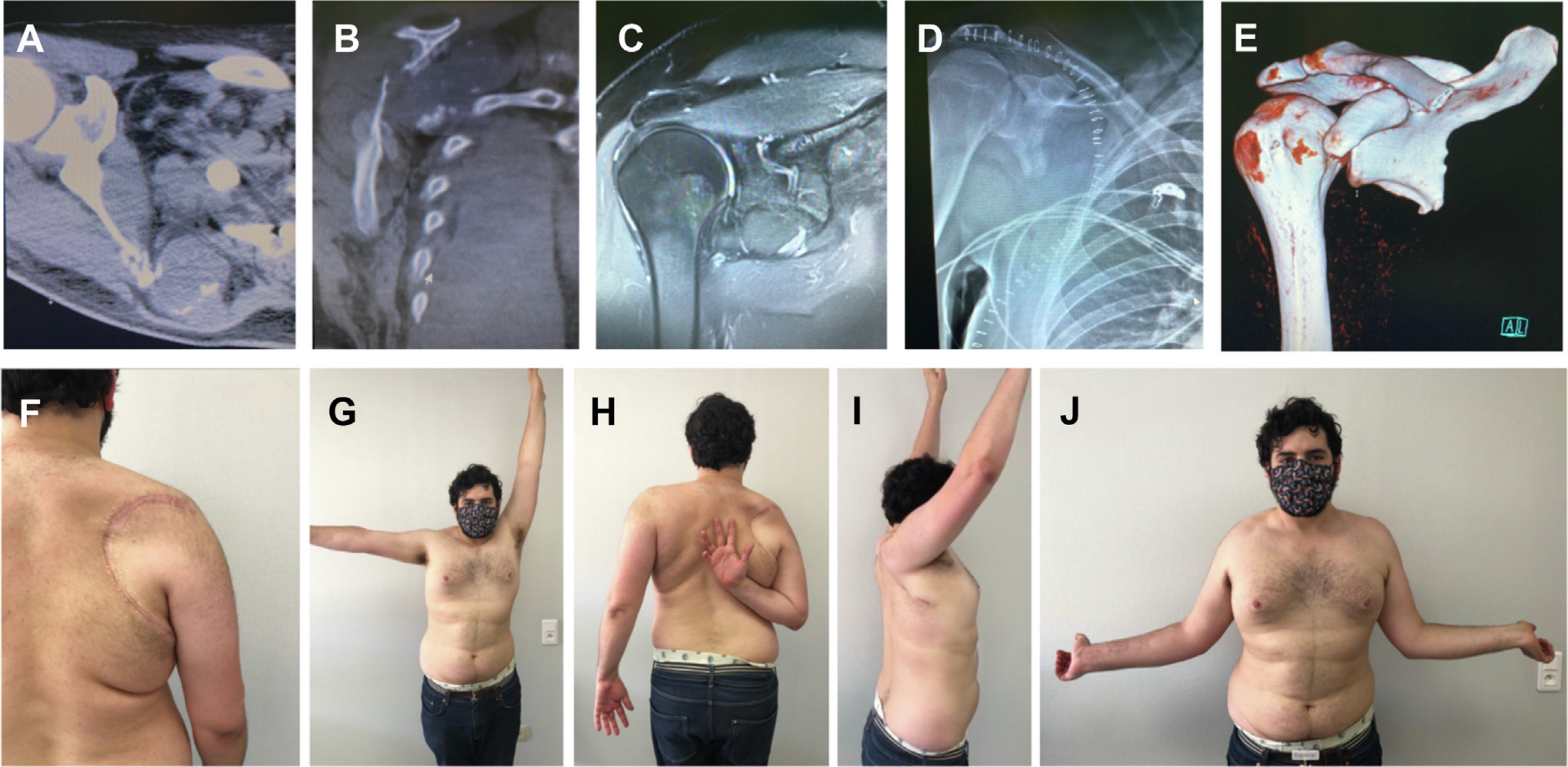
Example of partial scapulectomy. Possible glenohumeral conservation following a lesion of the body of the scapula seen in axial (A) and sagittal section on CT scan (B) as well as on frontal sections on MRI (T1, C) resection of the body of the scapula, the rhomboid and latissimus dorsi muscles. Radiological (D) and CT scan (E) findings postoperatively with the scar (F). At 7 years postoperative, here are the clinical results of this 31-year-old patient: abduction mobility (G), internal rotation (H), active anterior elevation (I), and external rotation (J). *MRI*, magnetic resonance imaging; *CT*, computed tomography.
